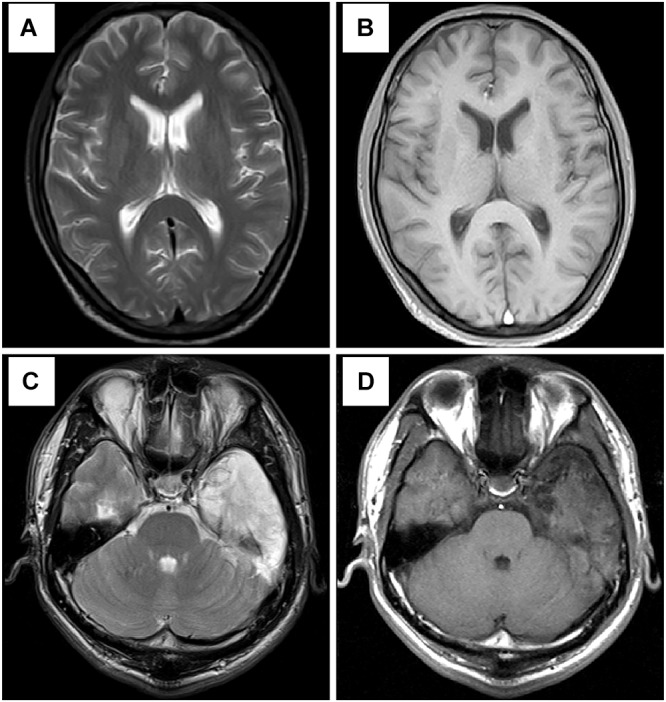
Results of head MRI. (A,B) Head MRI showed encephalatrophy in case 1. (C,D) Head MRI showed long T1 and long T2 signal intensities in the left temporal lobe, and enhanced MRI stated irregular light enhancement in case 2. (A,C) T2WI; (B,D) T1-weighted sequence after gadolinium enhancement.
